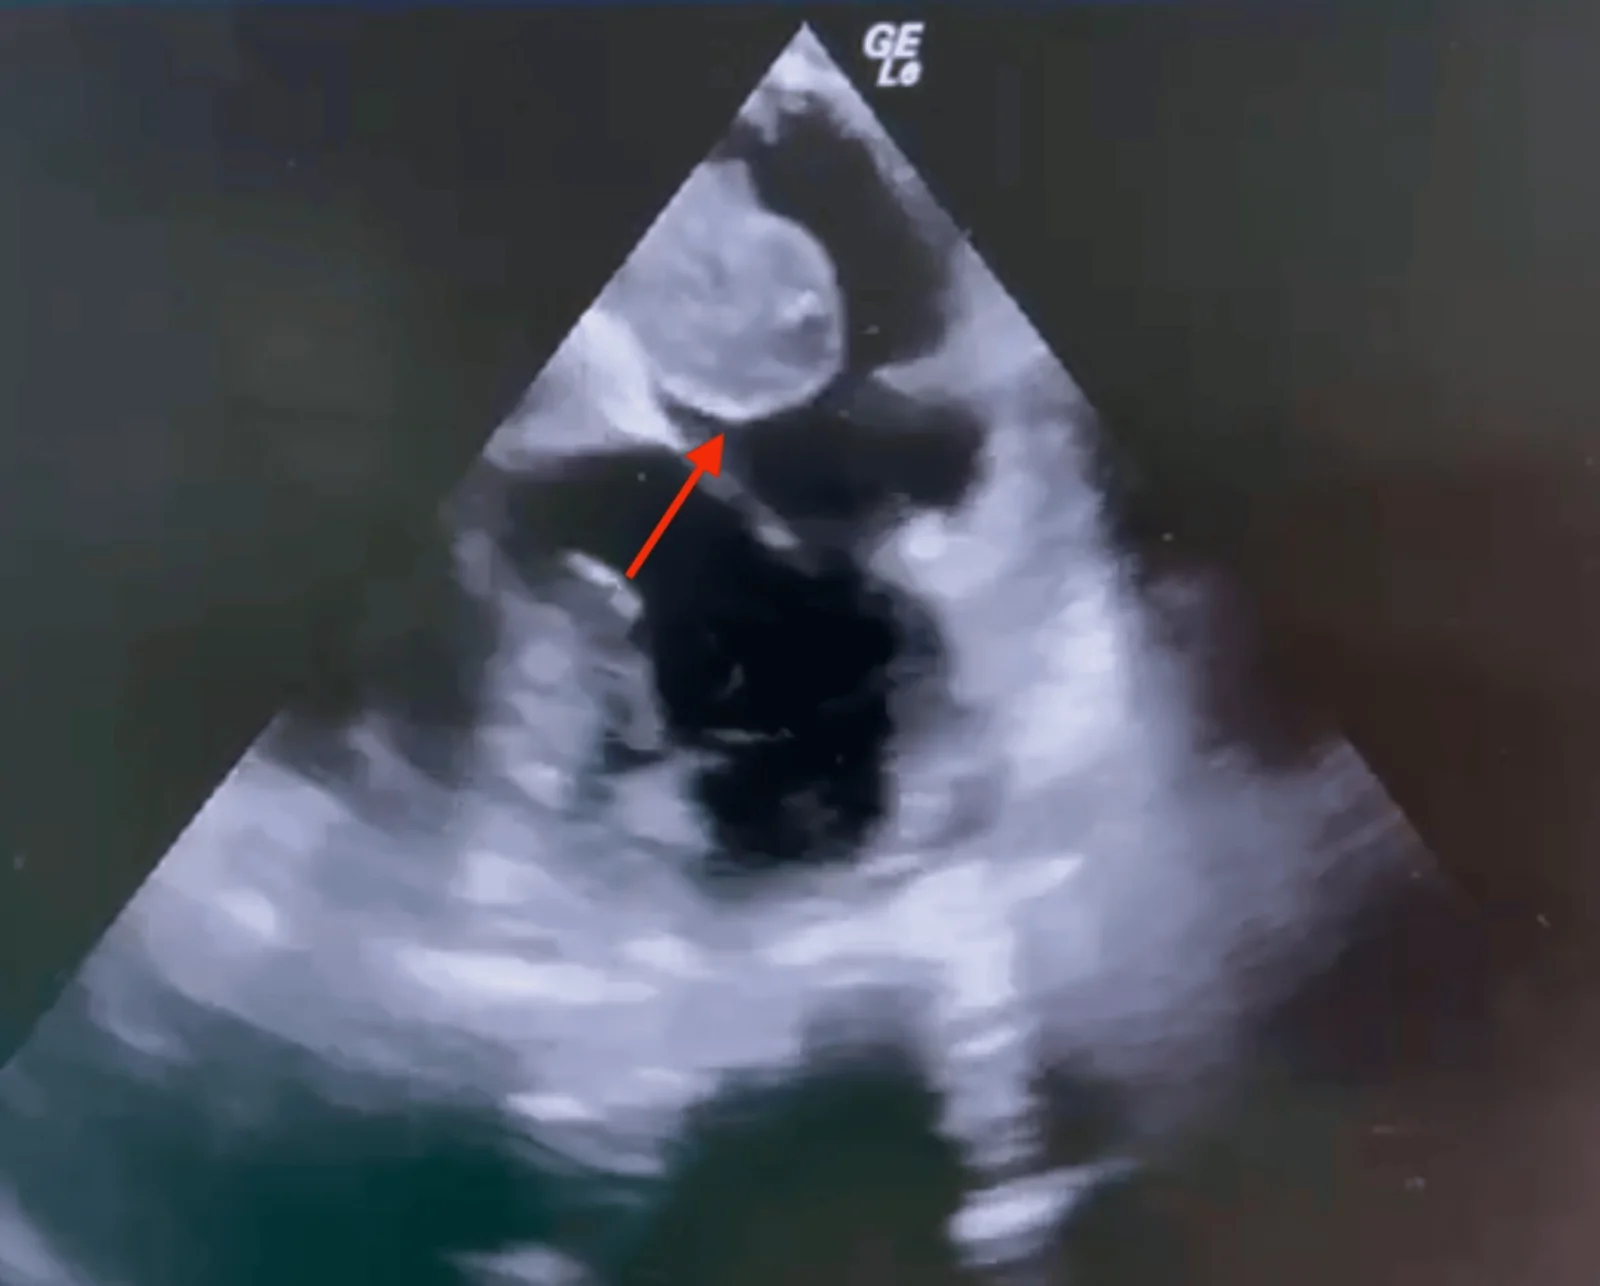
Transesophageal echocardiogram showing a well-circumscribed 3 × 2 cm echogenic left atrial mass consistent with a myxoma (arrow), attached by a narrow stalk to the interatrial septum at the fossa ovalis
